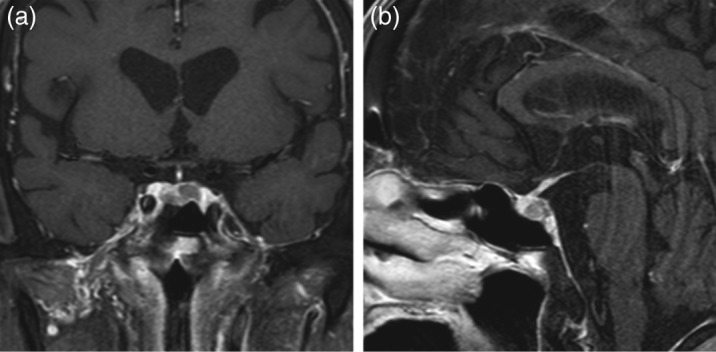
Coronal (a) and sagittal (b) T1-weighted MR images with gadolinium showing an intrasellar mass lesion with slight suprasellar extension.
